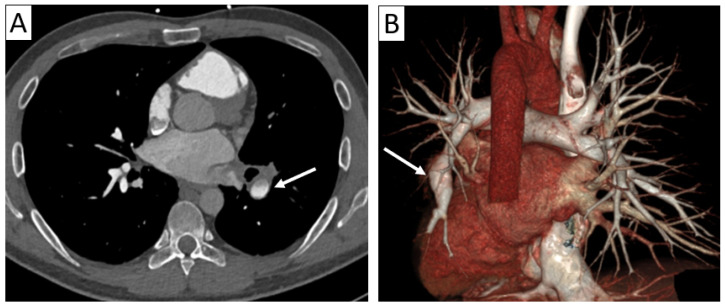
A 36-year-old male patient diagnosed with Hughes–Stovin syndrome presenting with hemoptysis and oro-genital aphthous ulcers with recurrent thrombotic events (inferior vena cava and renal veins). Axial thoracic CTA (A) with 3D-volume rendering (B) revealed a partially thrombosed left inferior lobar pulmonary artery aneurysm (arrows).
